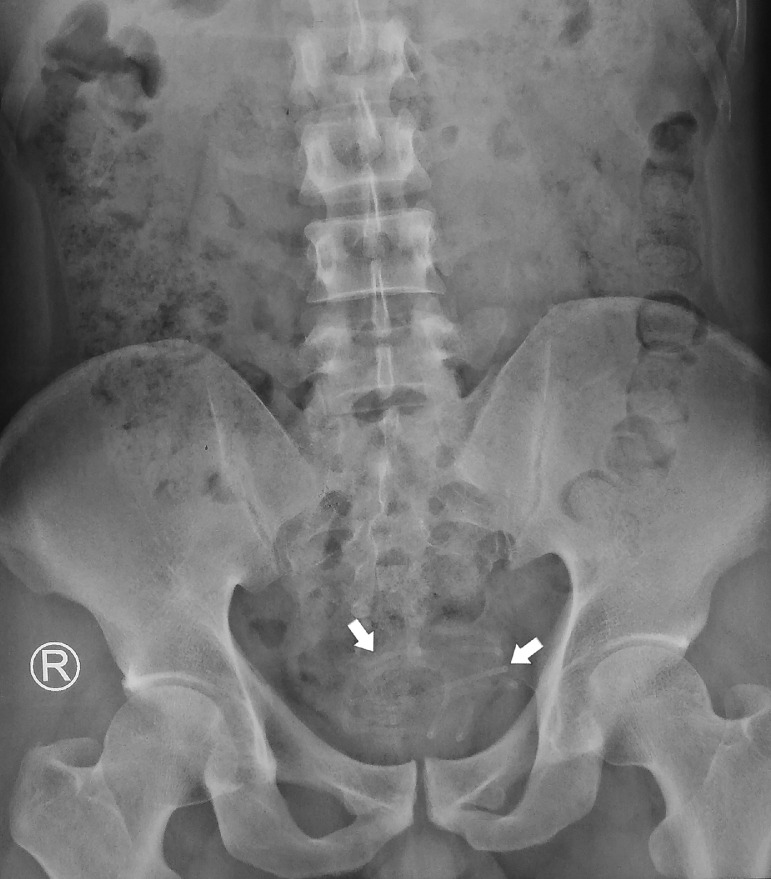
Plain pelvic X-Ray showing a semi-radio-opaque tubular shadow in the pelvis, as indicated by the arrows.
